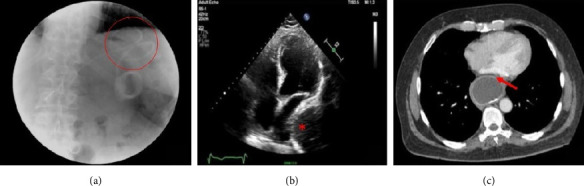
(a) Barium swallowing radiogram showing laparoscopic adjustable gastric banding rotation resulting in band slippage (red circle). (b) Transthoracic echocardiography, apical 4-chamber view showing left atrial compression from megaesophagus during diastole (red asterisk). (c) Computed tomography, transverse section showing megaesophagus causing extrinsic left atrial compression (red arrow) (adapted from De Silva et al.) [19].
